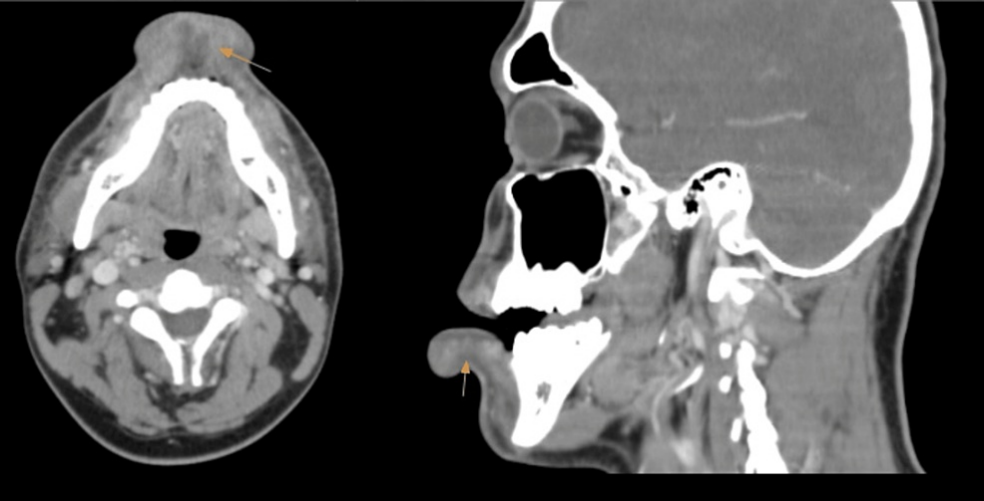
CT scan of the head and neck (sagittal on right side; axial on left side) There is a pronounced edema of the lower lip with a very limited edema of the upper lip. The associated peripherally enhancing collection extends to the margin of the gingiva (yellow arrows).
